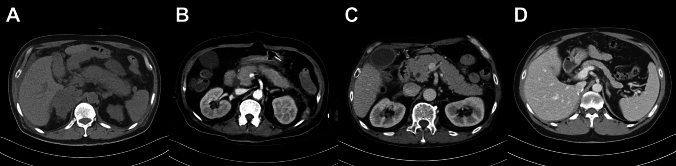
Representative imaging findings of ICI-related pancreatitis. A Contrast-enhanced CT showing diffuse pancreatic enlargement with peripancreatic fat stranding, consistent with acute interstitial pancreatitis pattern (80% of cases). B Focal pancreatic tail enlargement with subtle peripancreatic inflammation. C Mass-like enlargement of pancreatic head mimicking malignancy (AIP-like pattern). D Post-pancreatitis pancreatic atrophy on follow-up imaging
